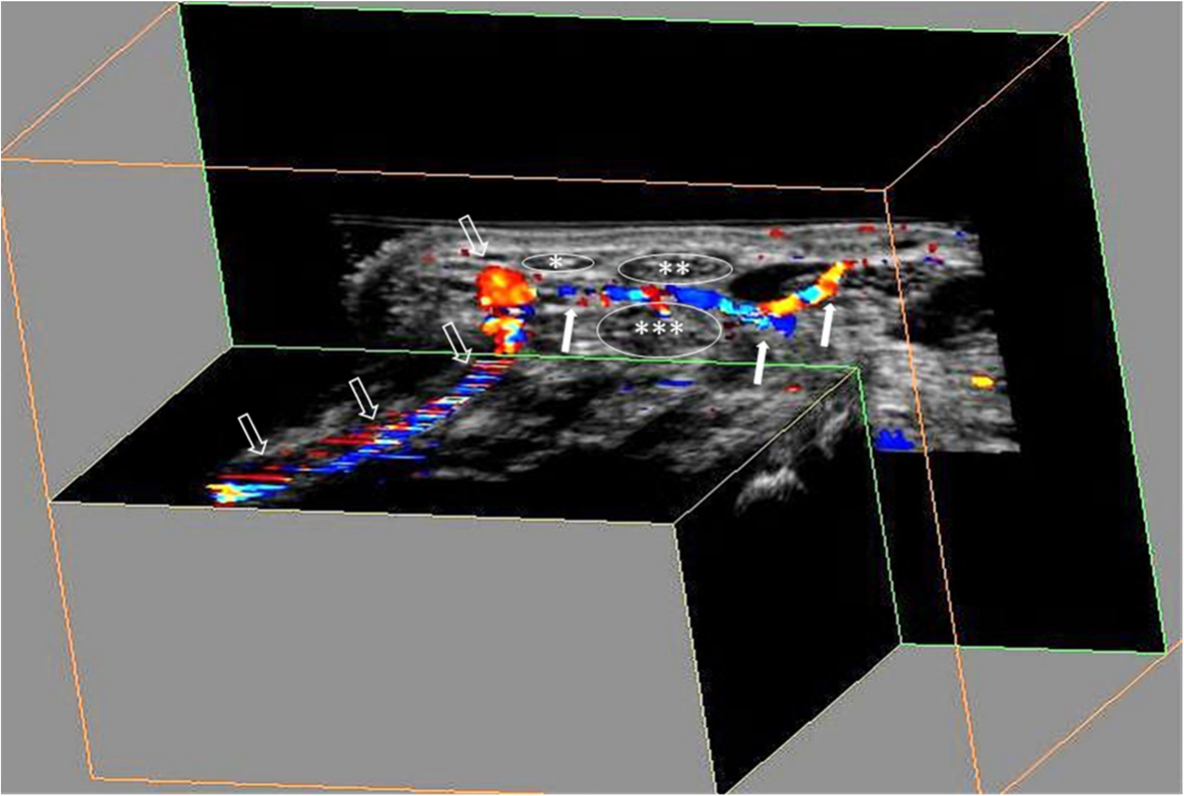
Ultrasound volume video clip of the distal part of the palmar wrist demonstrates the radial artery (open arrows) and a transverse feeding vessel (white arrows). Due to the close proximity to the tendon sheath of the feeding vessel, blooming or reverberation may be misinterpreted because they seem to be located inside the tendon sheath. *Insertion of the flexor carpi radialis; median nerve; *one part of the flexor digitorum profundus
